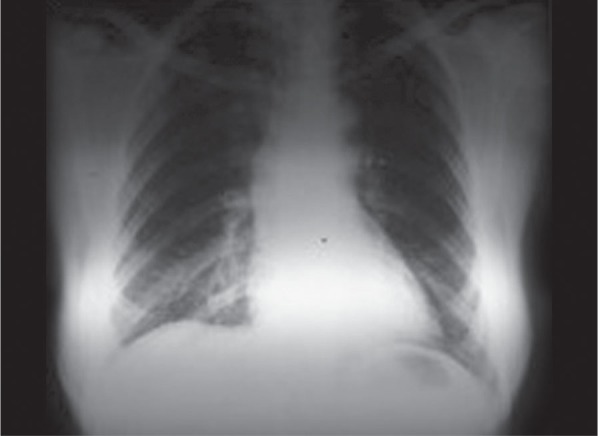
The chest radiography shows bilateral infiltrative appearance being prominent in the lower lobe of the right lung
